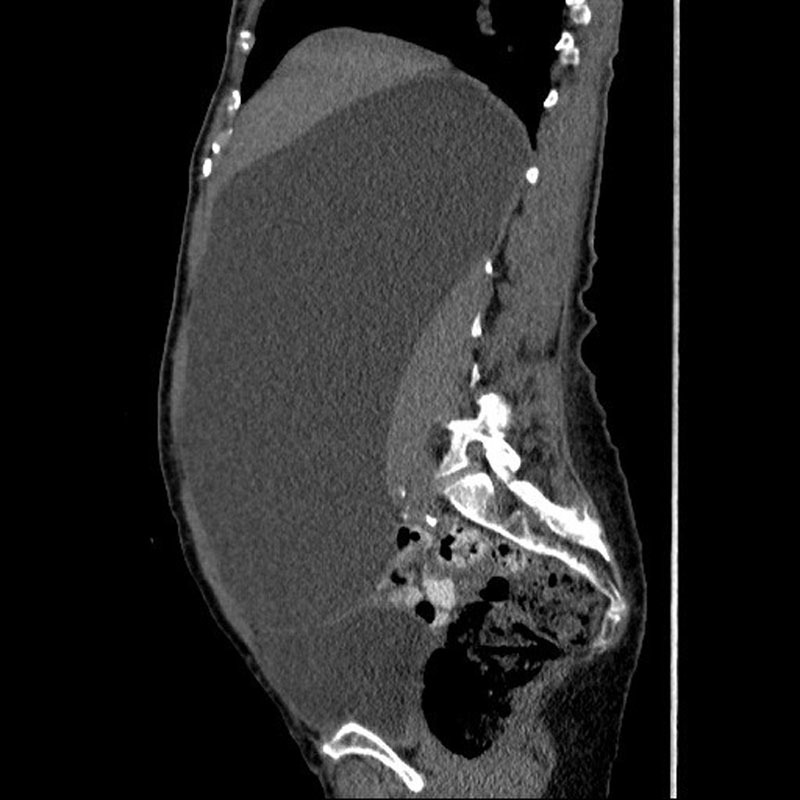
Abdominal computed tomography (sagittal plane) showing a giant retroperitoneal cyst.
